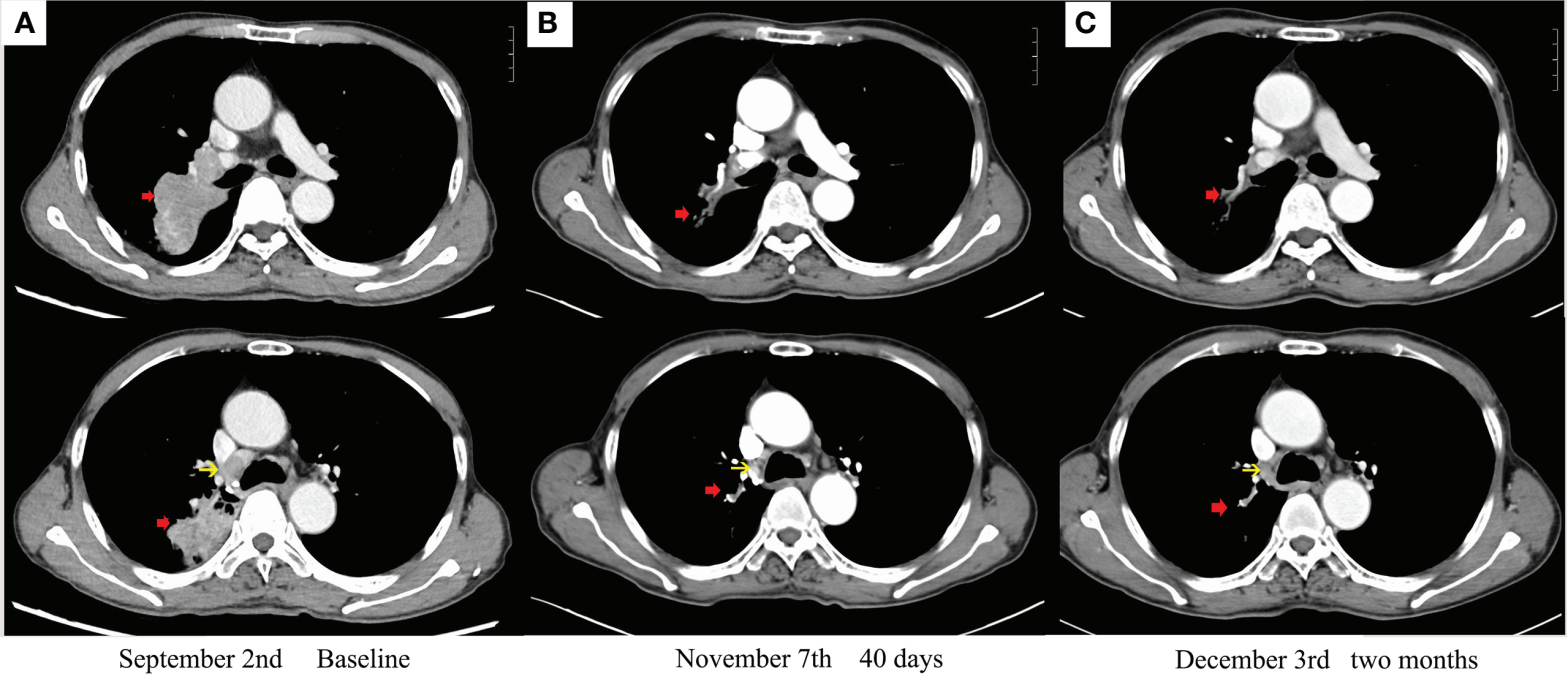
Enhanced chest CT scans during therapy: (A) baseline imaging. (B) After 40 days with savolitinib. (C) After 2 months with savolitinib. The red arrow indicates a primary tumor. The yellow arrow indicates metastatic lymph nodes.
